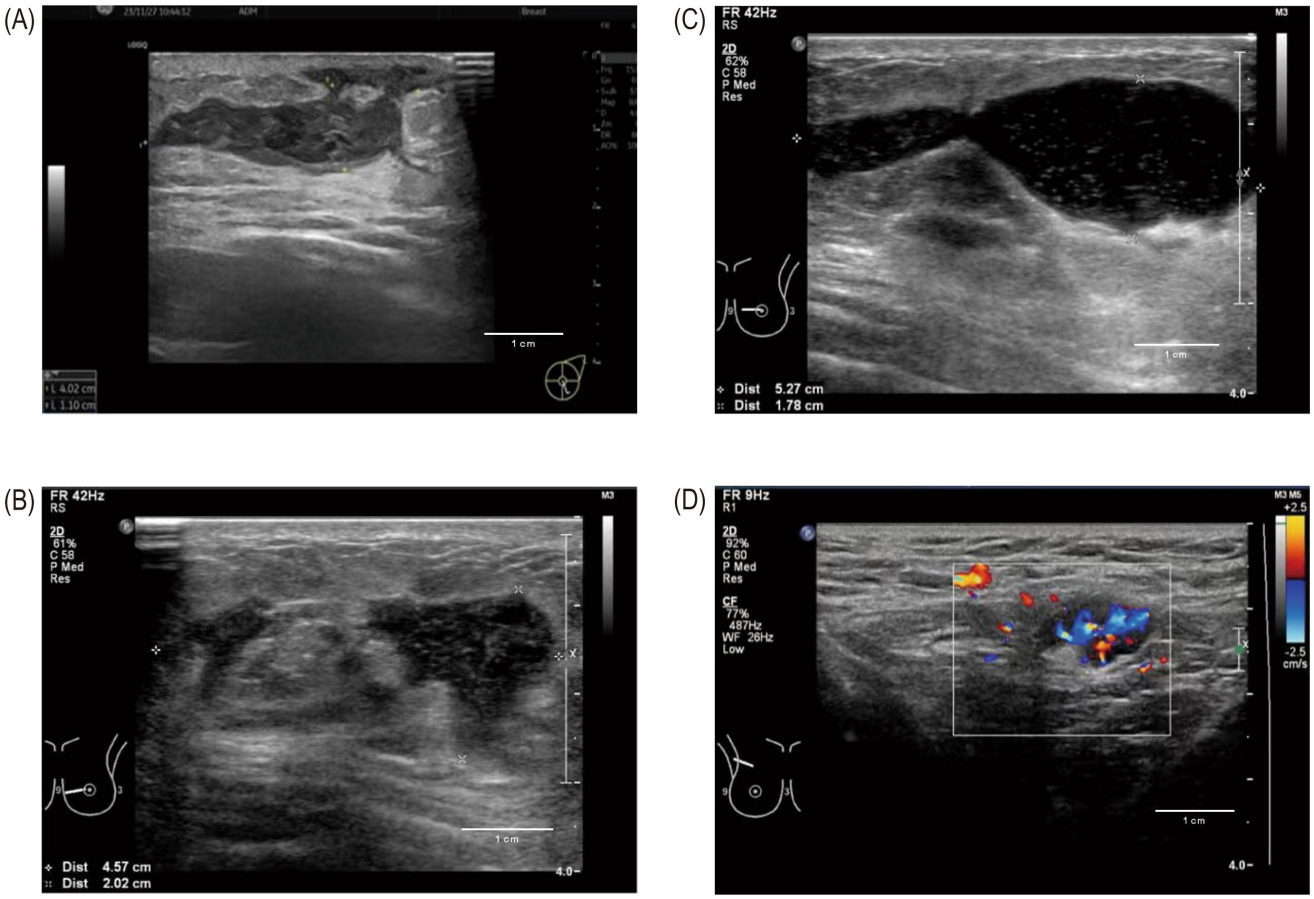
Typical ultrasound appearances in GM. (A) A central lesion showing an irregular, hypoechoic mass. (B) Multiple localized abscesses appearing as fluid-filled areas. (C) A fully developed abscess displaying a clear, fluid-filled space with enhanced through-transmission. (D) An enlarged axillary lymph node which shows a preserved fatty hilum and increased blood flow at its center.
